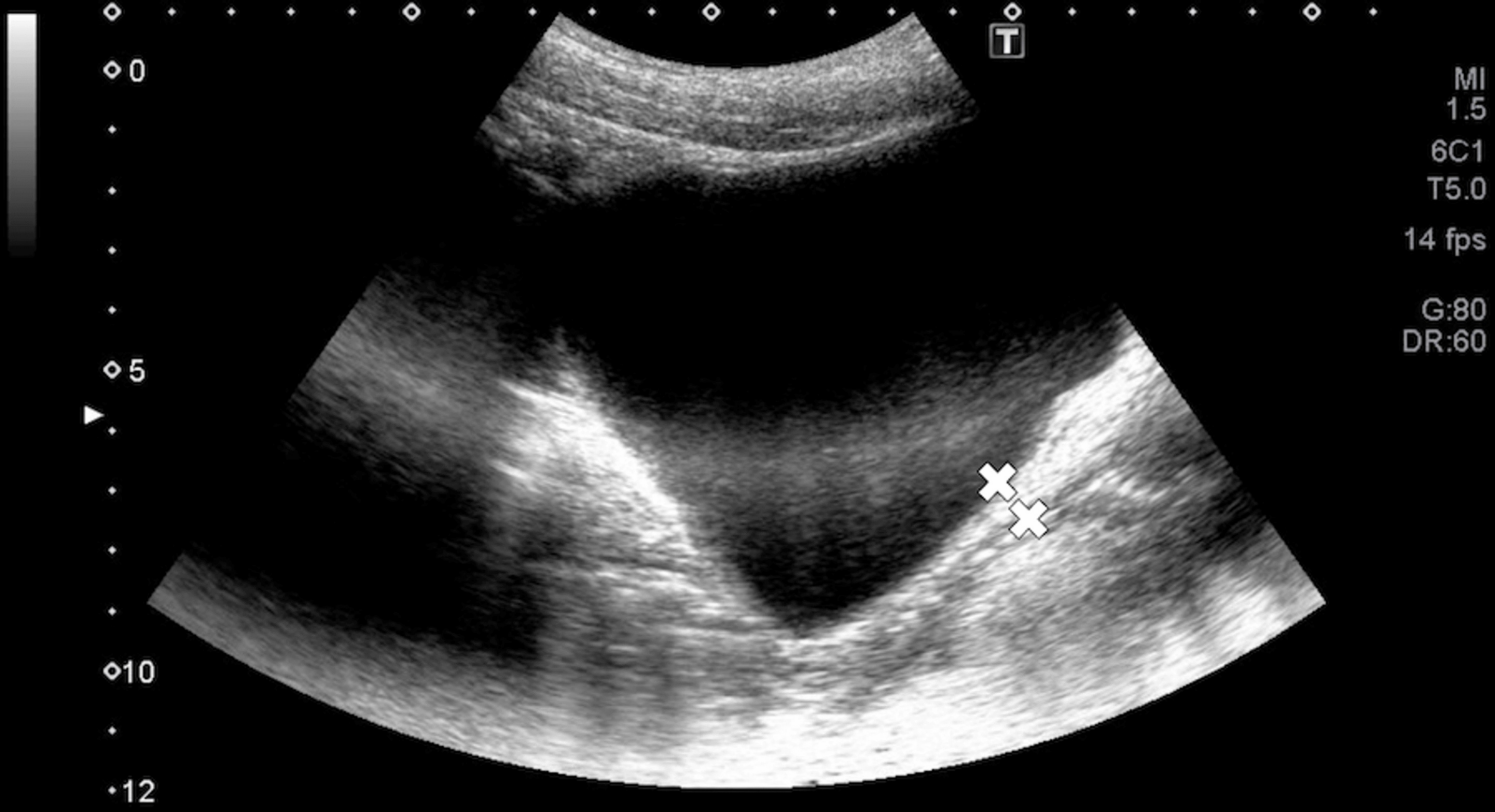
Ultrasound image of bladder base elevation during contraction (upper X mark indicates contraction, lower X mark indicates at rest) The point where the maximum displacement of the bladder base edge is clearly observed is designated as the reference point. At rest (a) and at maximum elevation (b), the reference point is indicated by “X.”
